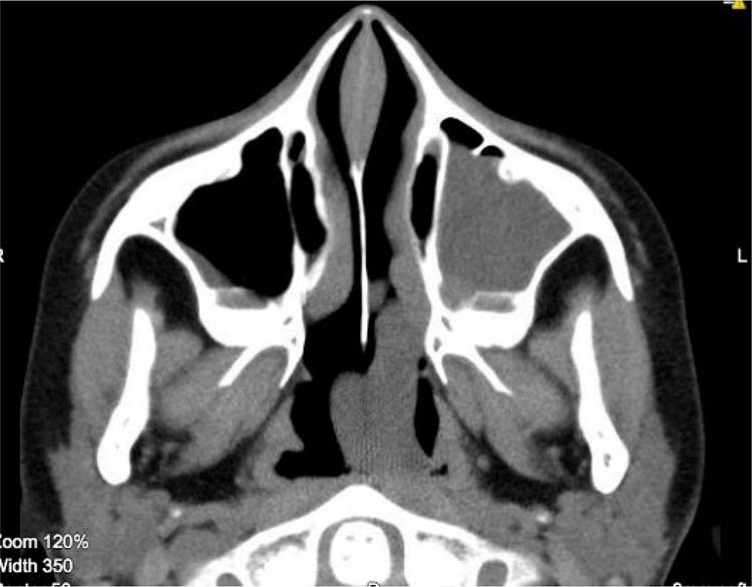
CT scan axial cut showing a large homogenous mass extending across the left nasal cavity.
